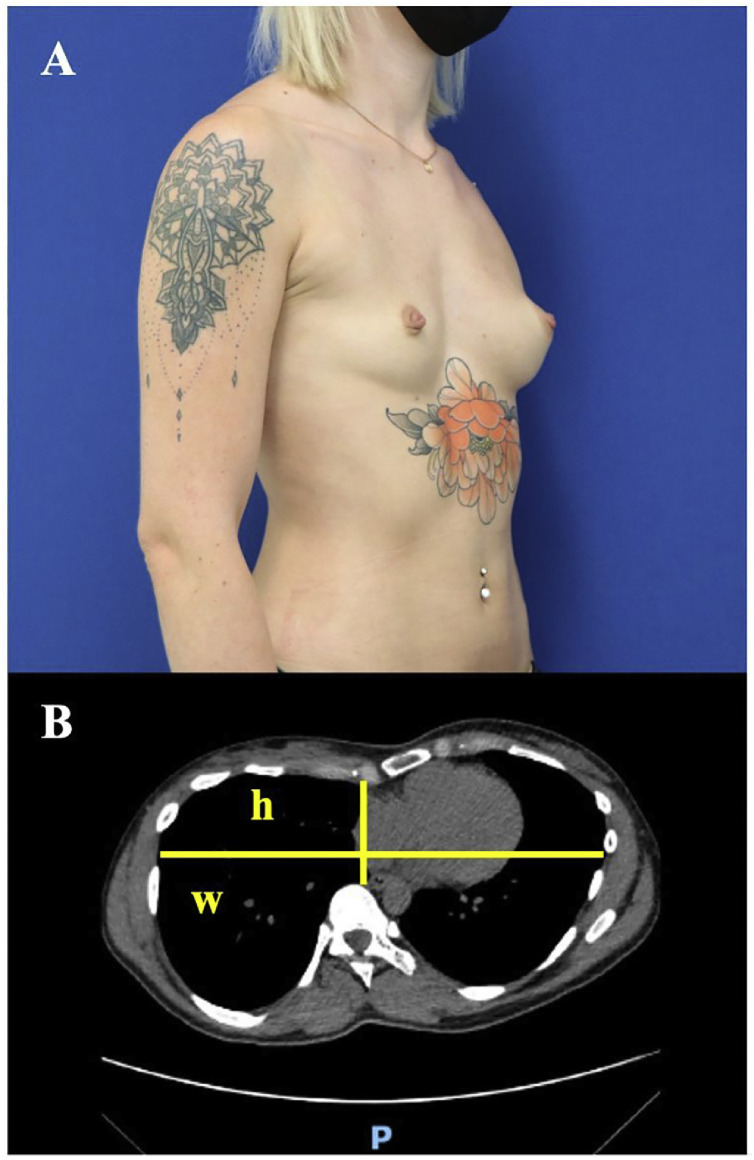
Pre-operative state. A: ¾ view of the funnel chest deformity that is more pronounced on the right side, enhancing the pre-existing volume asymmetry of the breast (right > left). B: CT scan section that shows the concavity at the caudal end of the sternum, resulting in an asymmetric deformity. Haller Index: w : *h* = 8.5 : 1.9 = 4.5.
